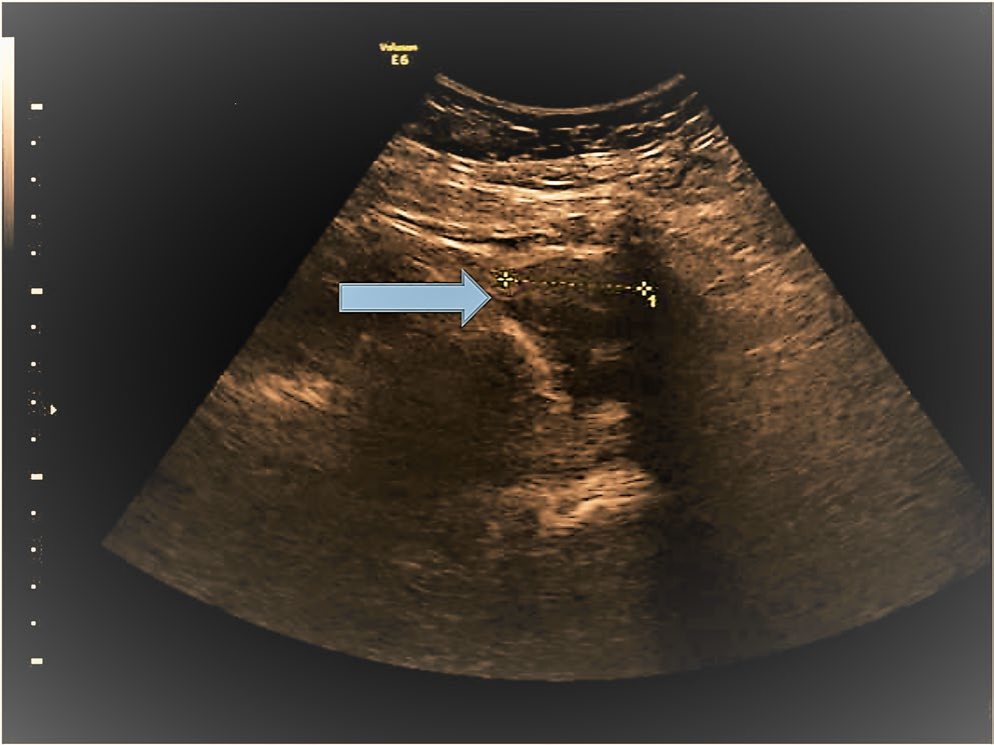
Hypogenic lesion underneath the scar of the abdominal wall with clear margins and benign characteristics via the transabdominal ultrasonography
